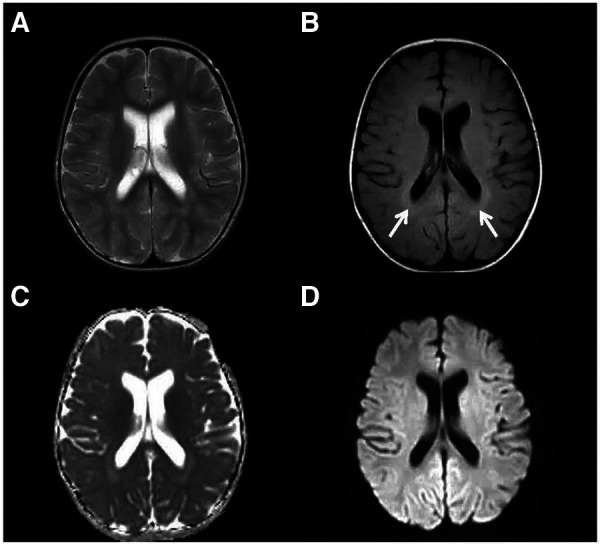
Elevated T2 FLAIR signal in the periventricular white matter. An axial T2-weighted (A), an axial FLAIR (B), a dADC (C) and a DWI (D) scans are shown and slightly elevated T2 FLAIR signal in the periventricular white matter can be seen (white arrow).
